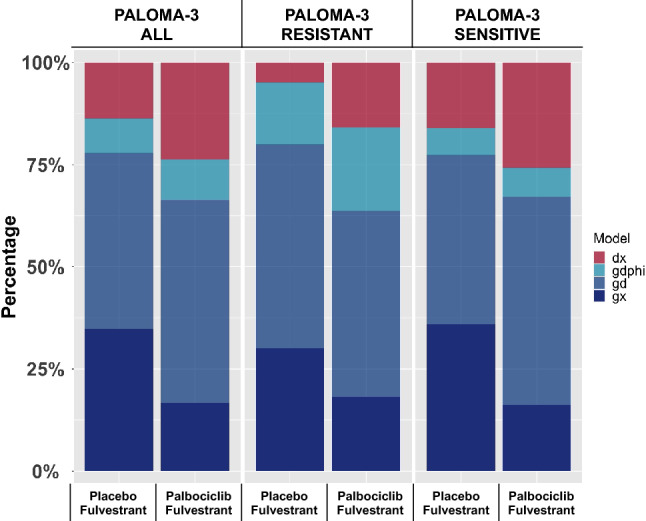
Distribution of equations best fit to radiographic tumor measurement data, by study treatment arm
